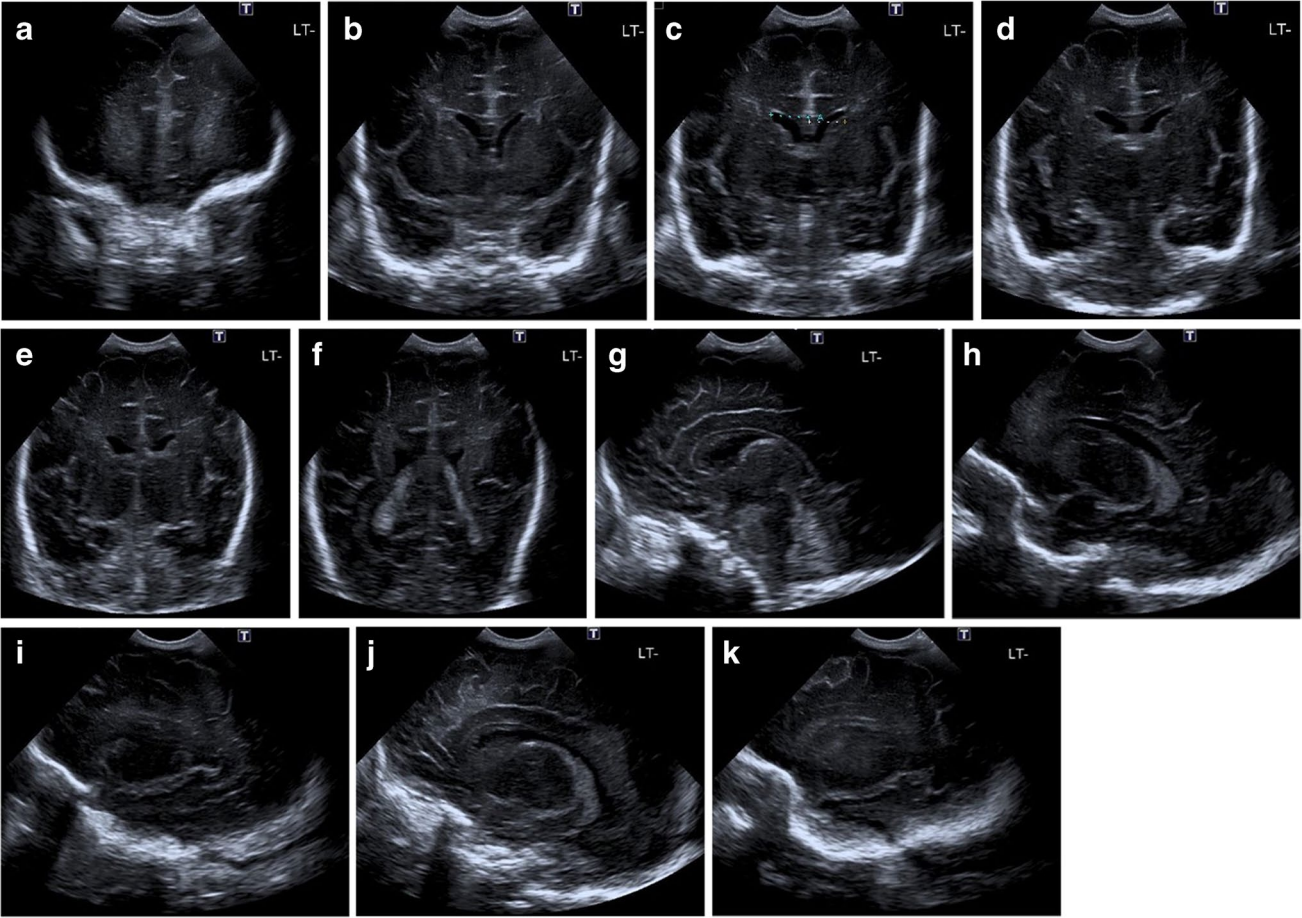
Standard normal 2-dimensional ultrasound views in a 41-day-old boy. Views show the frontal lobe (a), frontal horns (b), third ventricle and Sylvian with callipers measuring the bicoronal width of the frontal horns of the lateral ventricles (c), temporal uncus and Sylvian (d), tentorium (e), choroid plexus (f), midline (g), right caudothalamic groove (h), right temporal lobe (i), left caudothalamic groove (j), and left temporal lobe (k)
